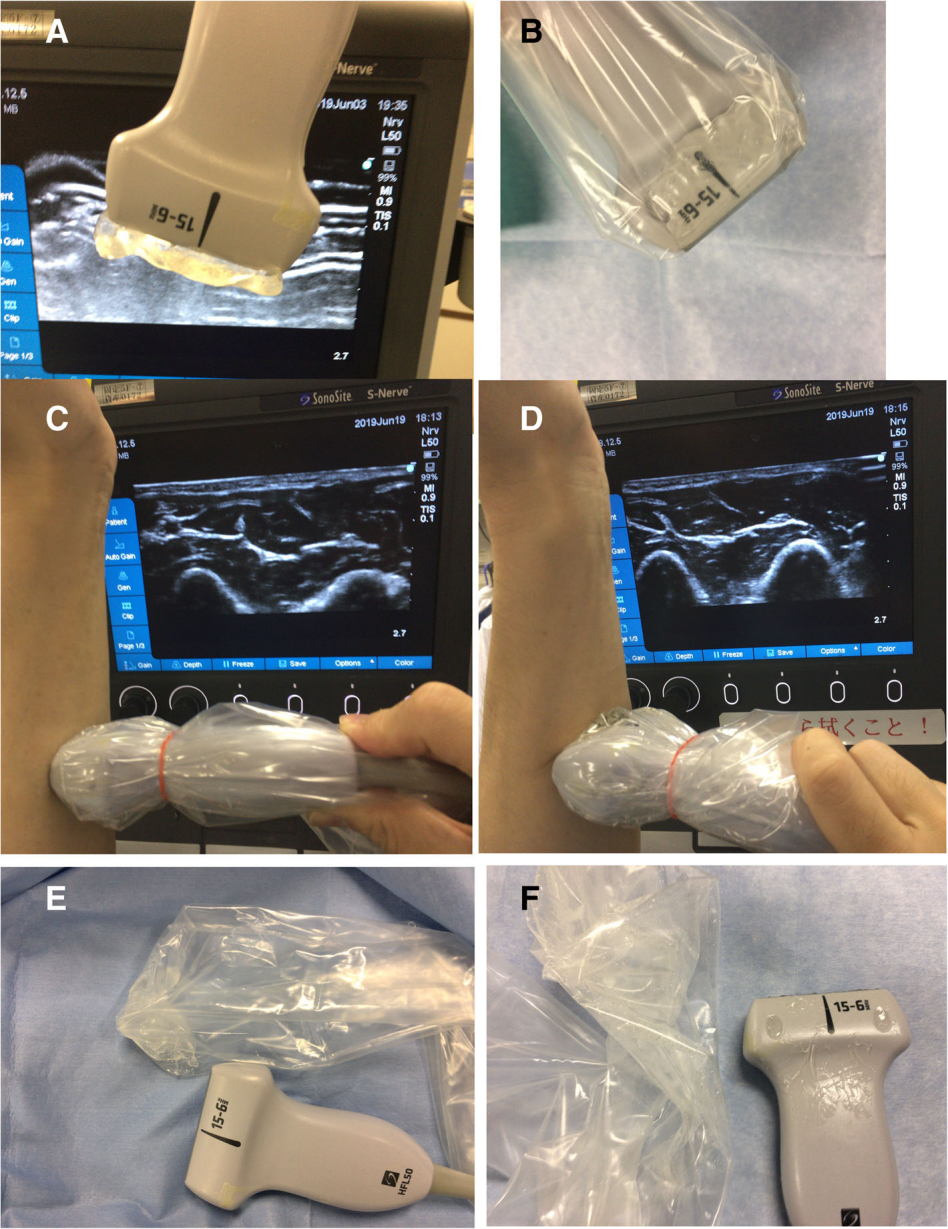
a Simple application of SLIME on the probe allows the SLIME to be placed firmly on the probe. b Application of SLIME on the probe allows entry of air between the probe and probe cover to be avoided. c The ultrasound image of the median nerve at the forearm obtained with the use of SLIME on the probe. d The ultrasound image with the use of water-soluble gel. e The probe remains smooth after removal of the probe cover using SLIME. f The probe after removal of the probe cover using gel
